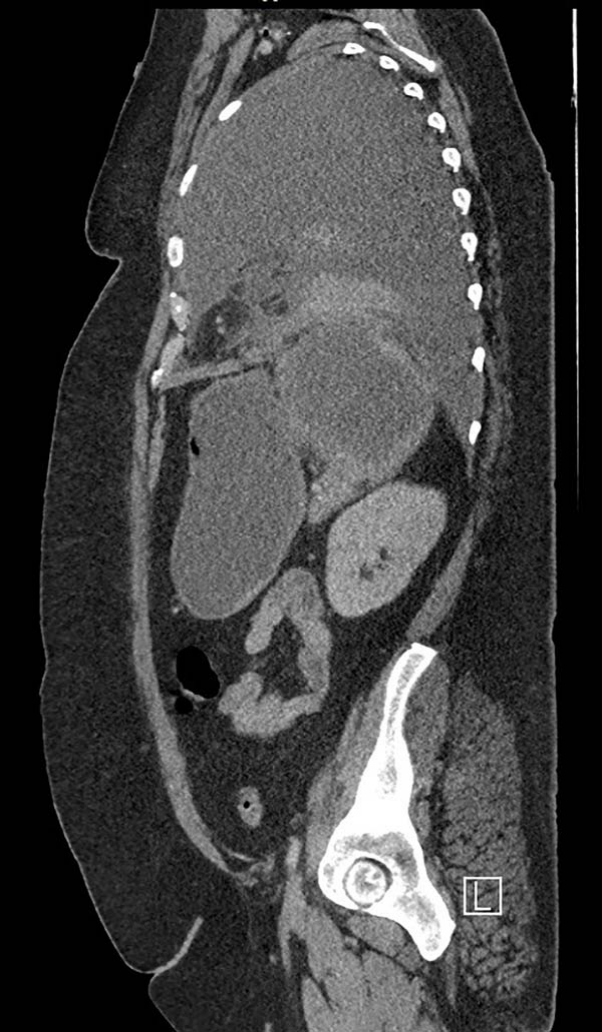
CT chest, abdomen and pelvis sagittal view: left-sided empyema and splenic abscess.
